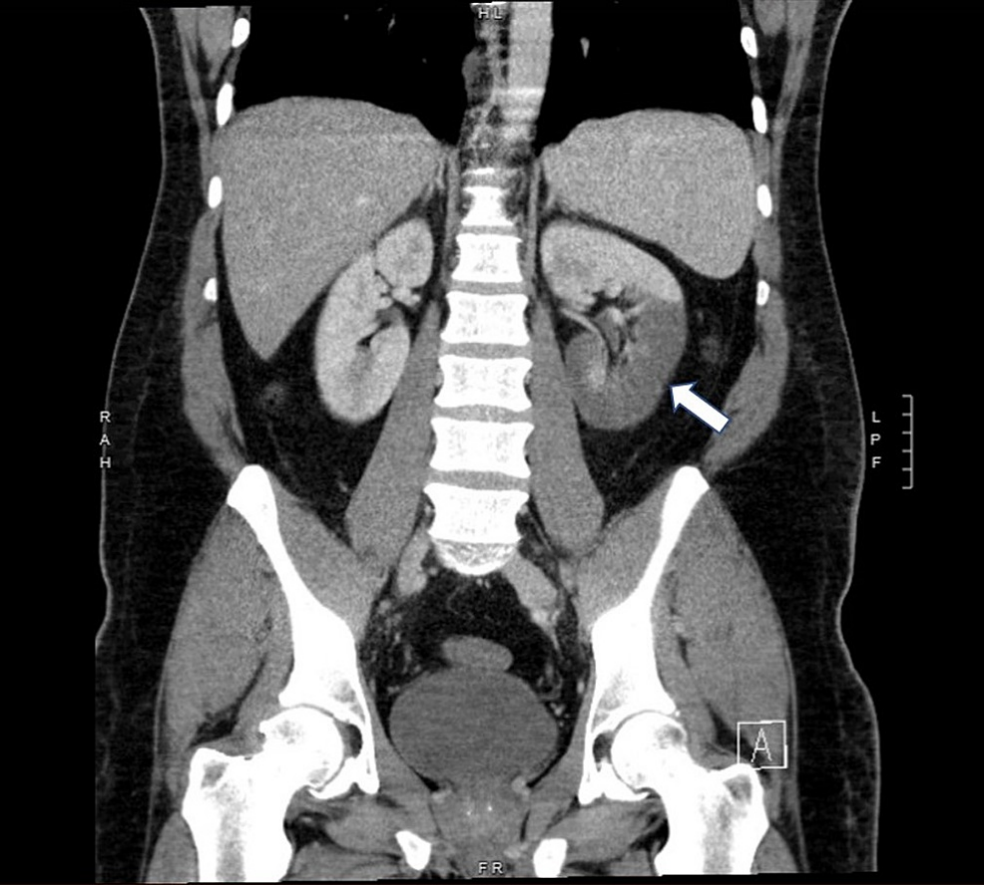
Coronal computed tomography scan of the abdomen with contrast showing reduced enhancement of the lower pole of the left kidney consistent with infarction.
